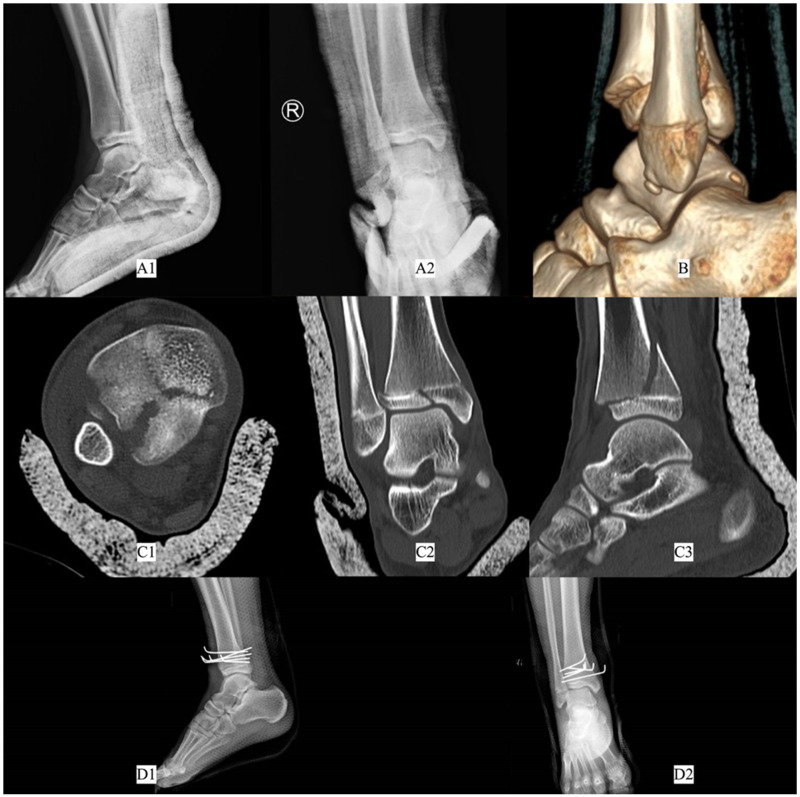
(A) initial x-ray after closed reduction. (B) Three-dimensional reconstruction CT after closed reduction. (C) Axial, coronal and sagittal image of initial CT after closed reduction. (D) Final radiography after surgery.
